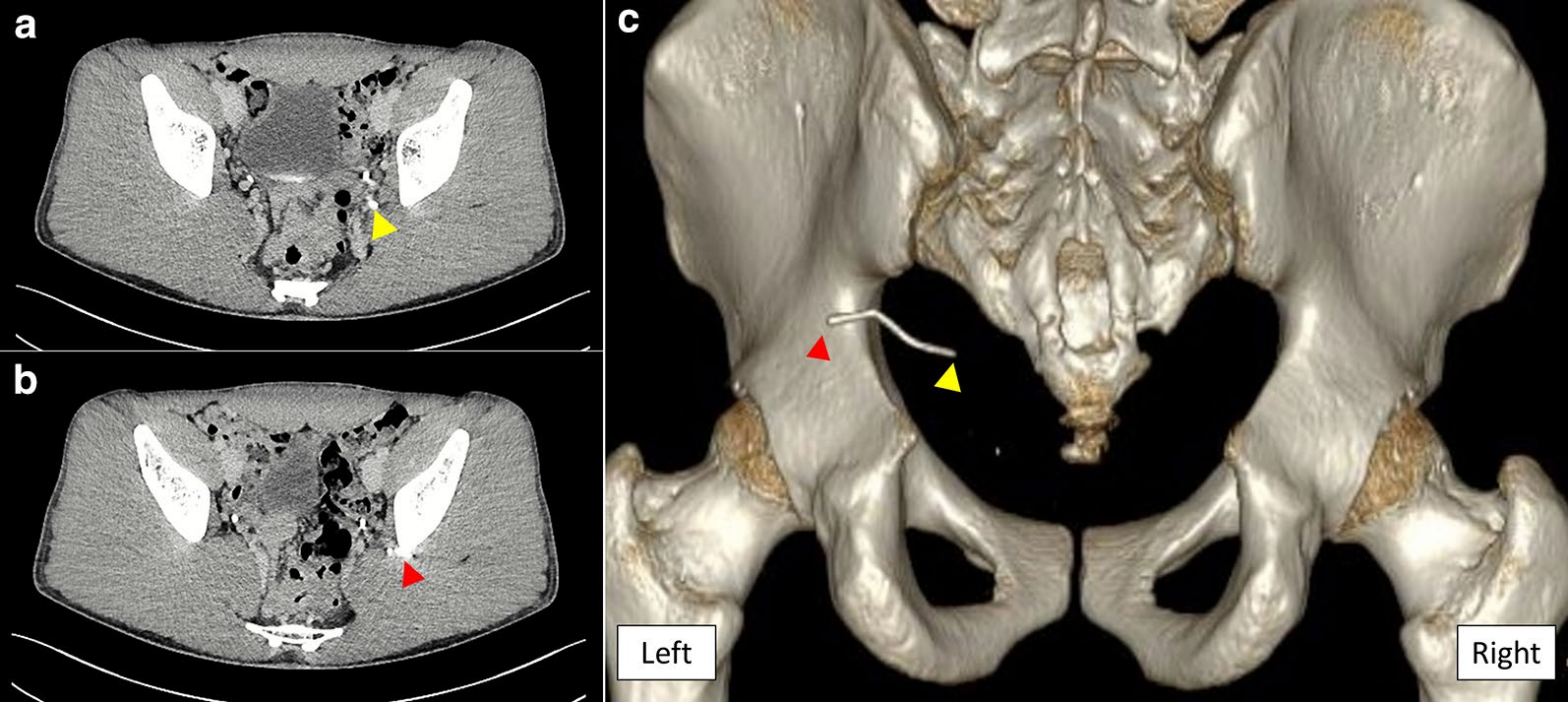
Computed tomography of the abdomen. a The tip of the needle (blue arrowhead) seems to reach the pelvic cavity through the retroperitoneum. b The stump of the needle (red arrowhead) is located in the gluteus. c Three-dimensional reconstructed image seen from the back clearly shows a linear, hyperdense foreign body in the gluteus
